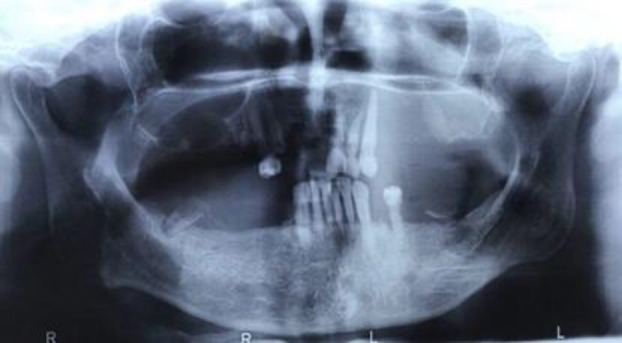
Panoramic radiograph showing maxillary left sinus involvement
